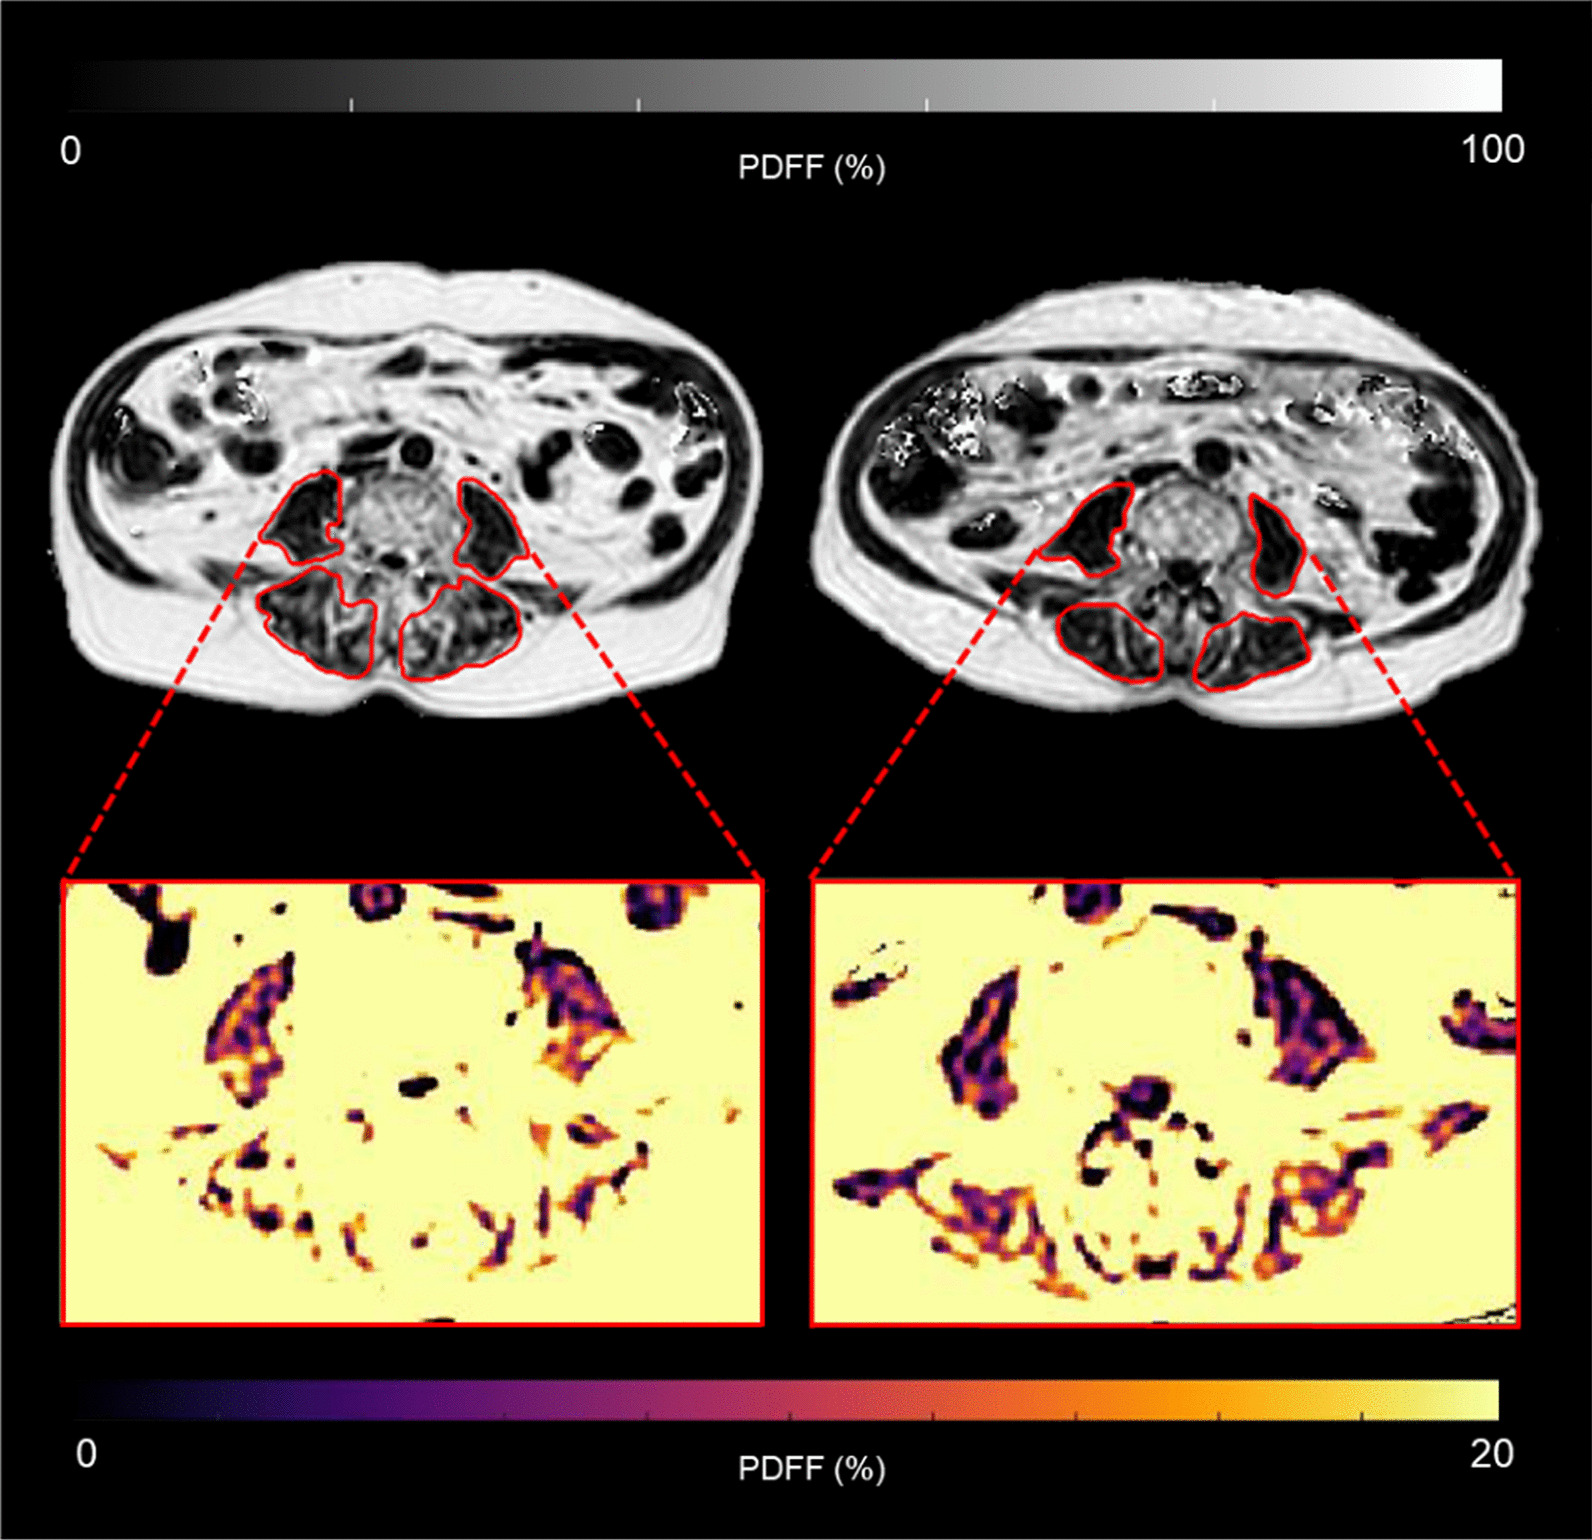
Skeletal paraspinal muscle changes in a patient with cancer cachexia: axial abdominal MRI scan of a 74 year-old patient with squamous cell carcinoma of the esophagus at baseline (left image) and follow-up after 335 days (right image). Relative muscle volume change was − 19.9% for the erector spinae and − 26.3% for the psoas muscle. Relative change of contractile tissue volume was − 19.4% in erector spinae muscle and 25.3% in psoas muscle. Relative change in fat volume was − 21.2% in erector spinae muscle and 27.7% in psoas muscle. Absolute PDFF (%) difference was 0.5 in erector spinae muscle and 1.3 in the psoas muscle. BMI decreased from 24.1 to 21.1 kg/m^2^. *BMI* body mass index,* PDFF* proton density fat fraction
